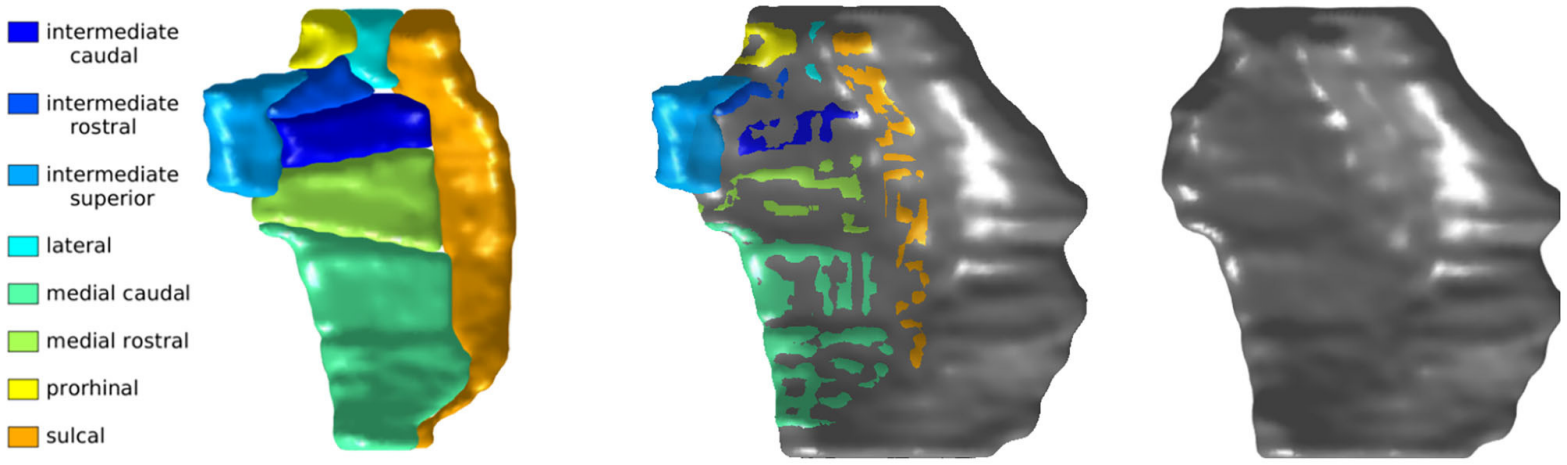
Krimer subregions of ERC labeled on 11T *ex vivo* MRI (left). ERC plus TEC labeled on same MRI following protocol described in section 4.2 (right). Overlay of segmentations (middle). The orientation is as follows: anterior (top), posterior (bottom), medial (left), lateral (right).
